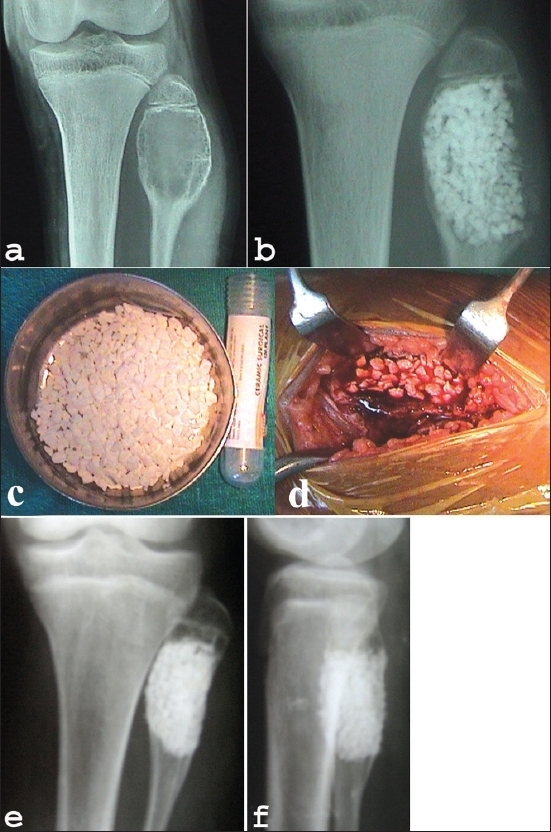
(a) Preoperative x-ray of knee AP view (a) shows a cavitary lesion (aneurysmal bone cyst) of upper end of fibula in 14 year old girl. Immediate postoperative x-ray (b) shows the cavity filled with HA granules. (c) and granule filled cavity (d) Peroperative clinical photograph of same patient showing HA granules (d). (e) Anteroposterior and lateral x-rays (f) of same patient at 2 yrs follow-up shows remodelling.
